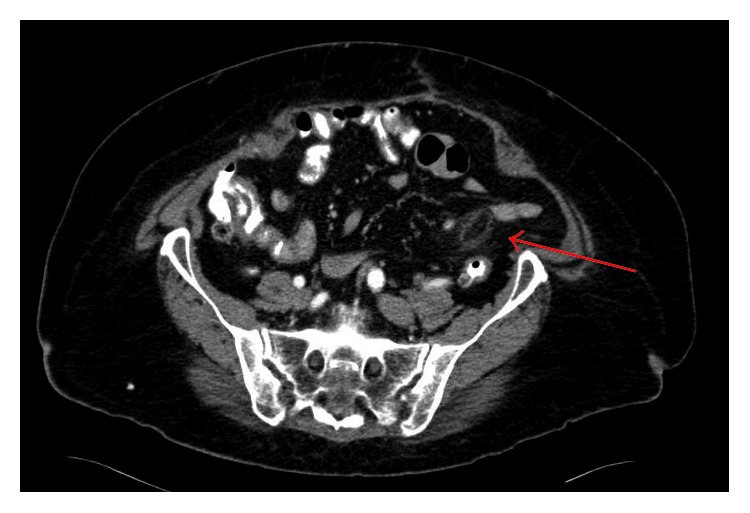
CT findings consistent with epiploic appendagitis including an ovoid fat density structure with surrounding peripheral inflammatory change (red arrow).
